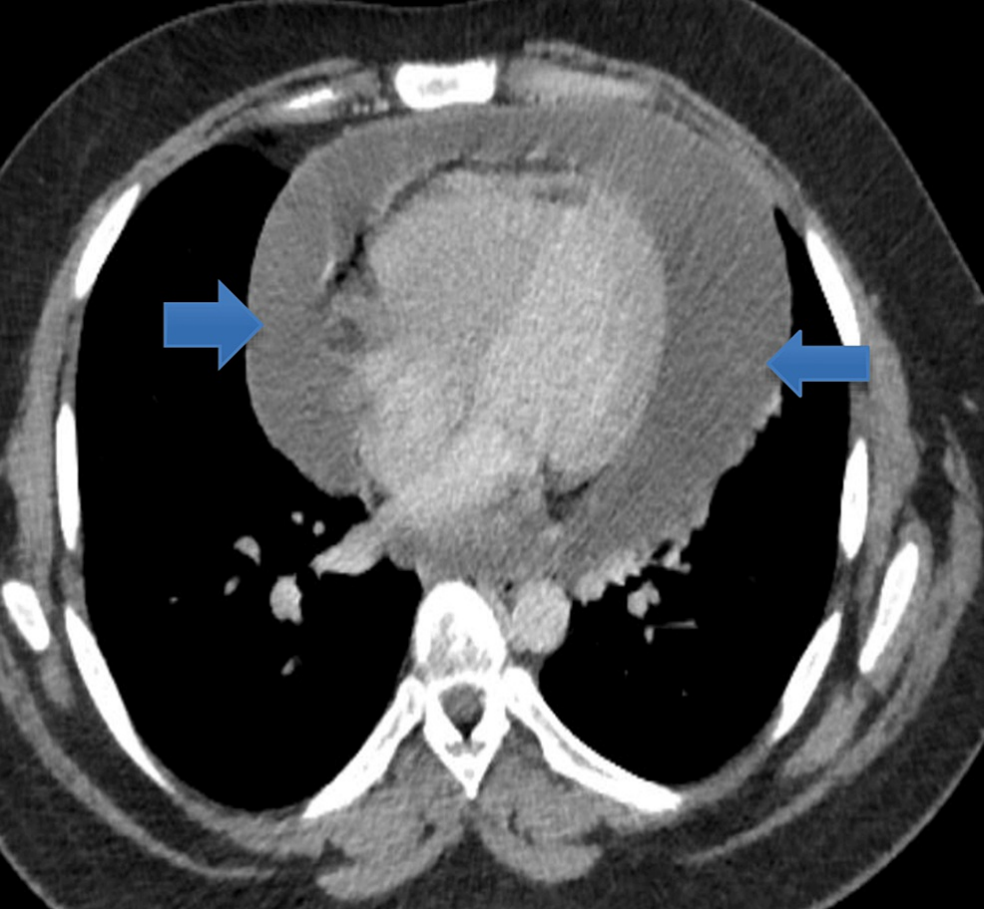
Computed tomography scan of the abdomen and pelvis showing a large pericardial effusion (blue arrows).
